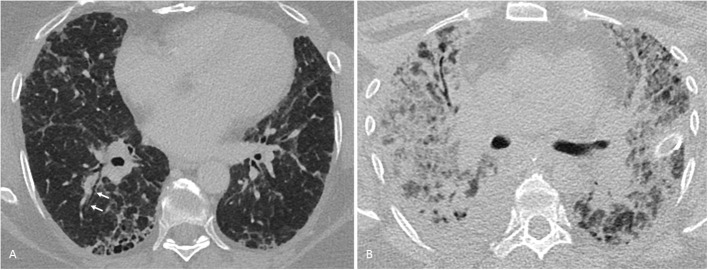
Interstitial lung disease in a 56-year-old woman with rheumatoid arthritis. (A) One millimeter transverse axial CT-section through the lung bases show subpleural honeycombing and early traction bronchiectasis (arrows), consistent with a usual interstitial pneumonia pattern. (B) Nine months later, the patient developed severe dyspnea at rest and required mechanical ventilation. On bronchoalveolar lavage, influenza A virus was found to be present. A follow-up CT now showed a small right-sided pleural effusion and multifocally confluent consolidation, partially obscuring equally patchy bilateral ground-glass opacification. A few thickened septae (crazy-paving pattern) could be delineated (not shown). These findings were consistent with a viral pneumonia. Despite extracorporeal membrane oxygenation therapy, the patient deceased.
